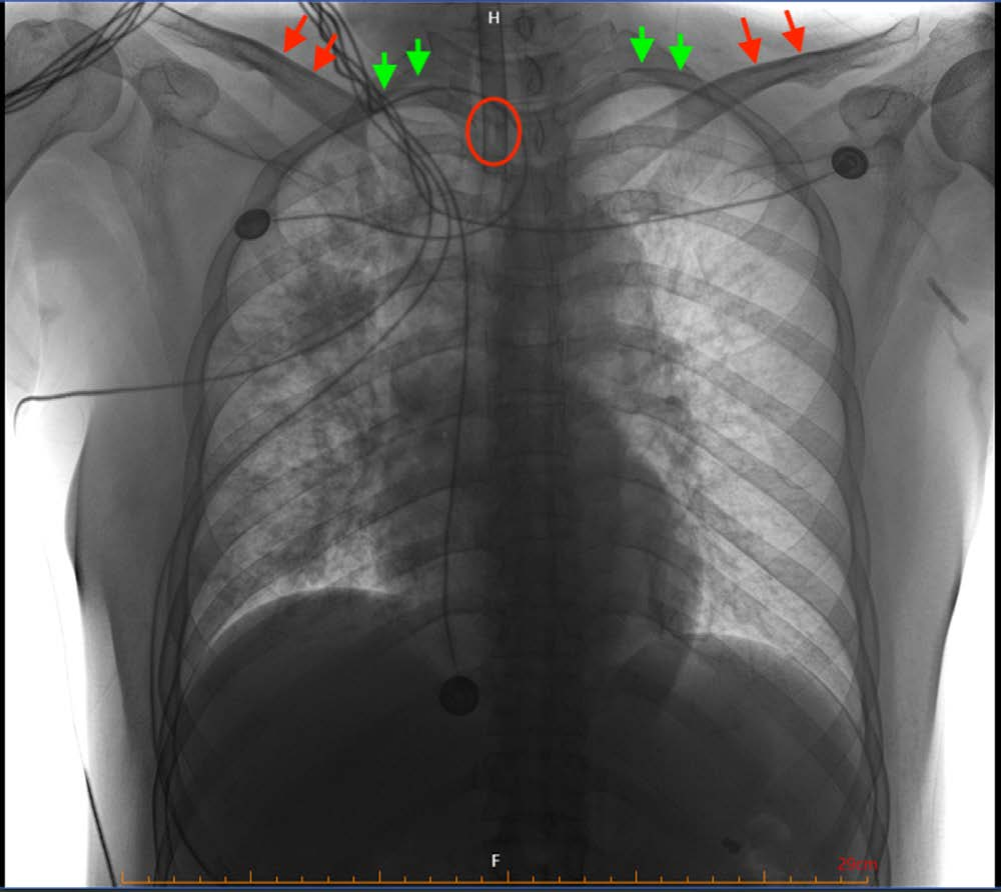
Chest radiography shows that the position of endotracheal tube cuff (red circle) is below the thoracic inlet, which are defined by the first rib (green arrows) and clavicle (red arrows).^[9,10]^
